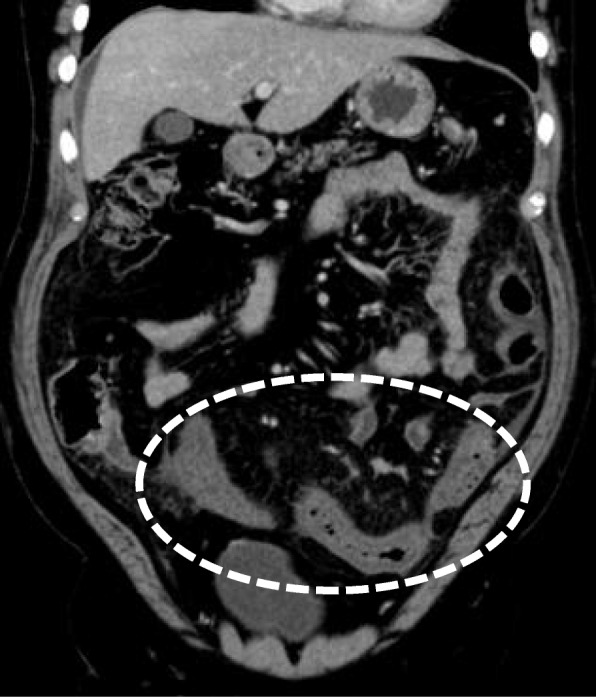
Portal venous-phase CT of the abdomen demonstrating marked wall thickening with poor mural enhancement and extensive submucosal edema in the sigmoid colon (white dotted circle)
